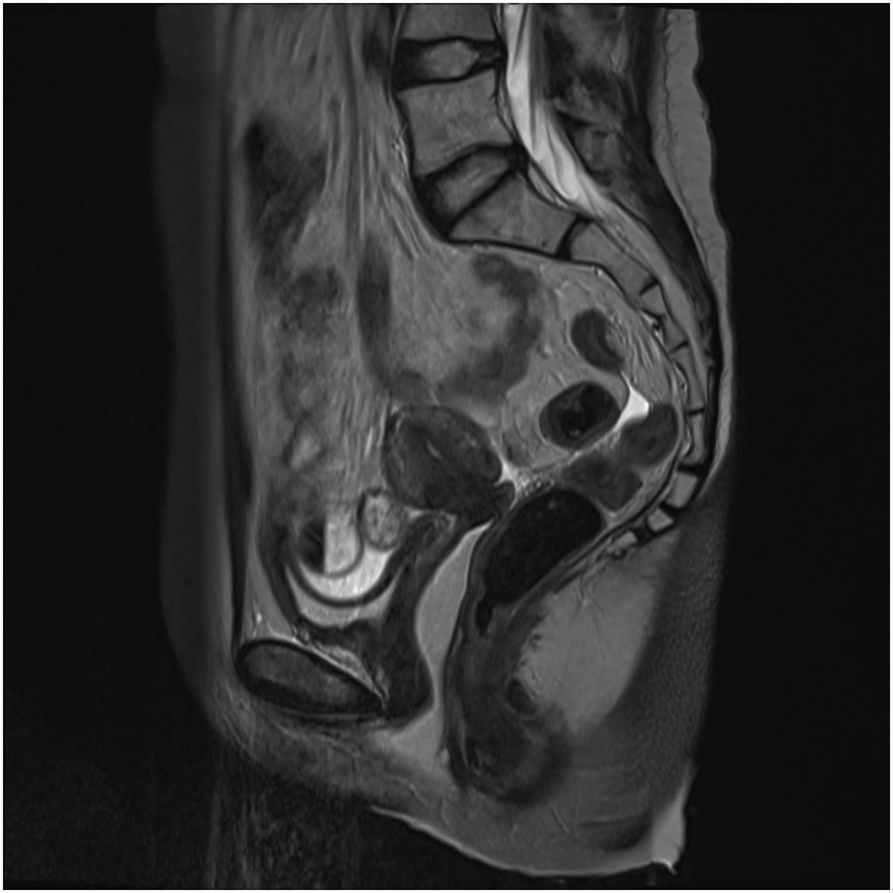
Patient #1: MRI 3 months after treatment of cervical necrosis (April 2009).
